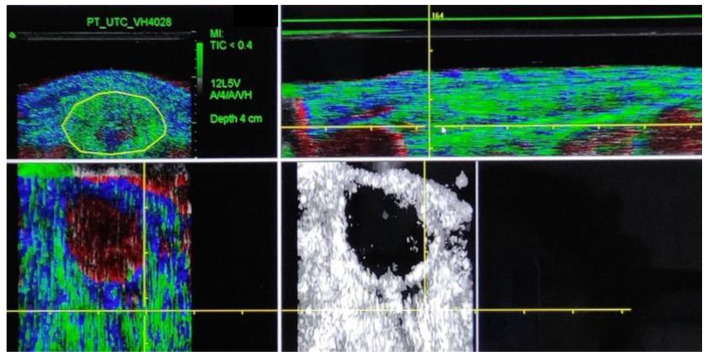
Ultrasound tissue characterization of the proximal tendon. Resection of the crossed sectional area at 20% tendon length. Color green, blue, red and black correspond to echo-type I, II, III, and IV respectively.
